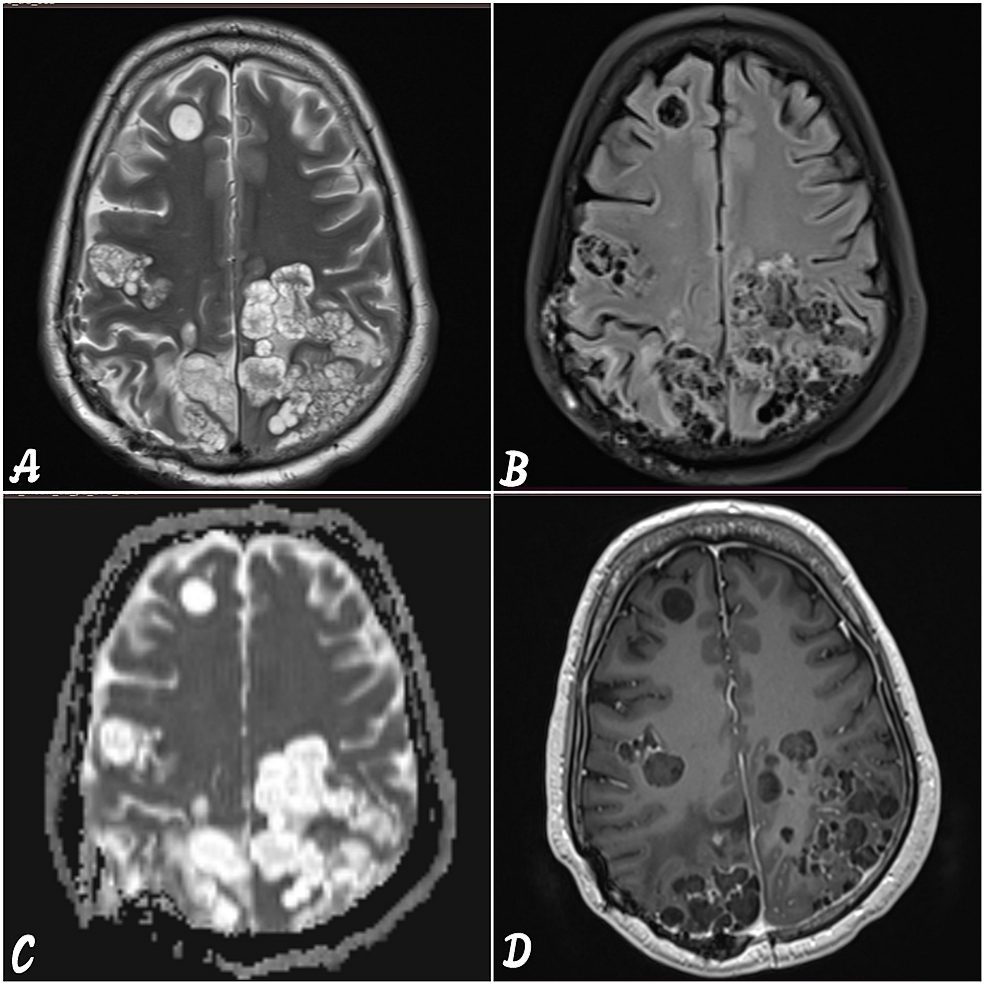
MRI brain with contrast (selected axial images). (A) T2-weighted image showing persistent cluster of thin-walled cystic lesions appearing hyperintense. (B) Fluid-attenuated inversion recovery (FLAIR) is showing hypointense signal in bilateral cerebral hemisphere (frontoparietal region and left occipital region). (C) Diffusion-weighted images (DWI) show no diffusion restriction. (D) T1 post-contrast image shows mild peripheral perilesional enhancement in bilateral cerebral hemispheres.
